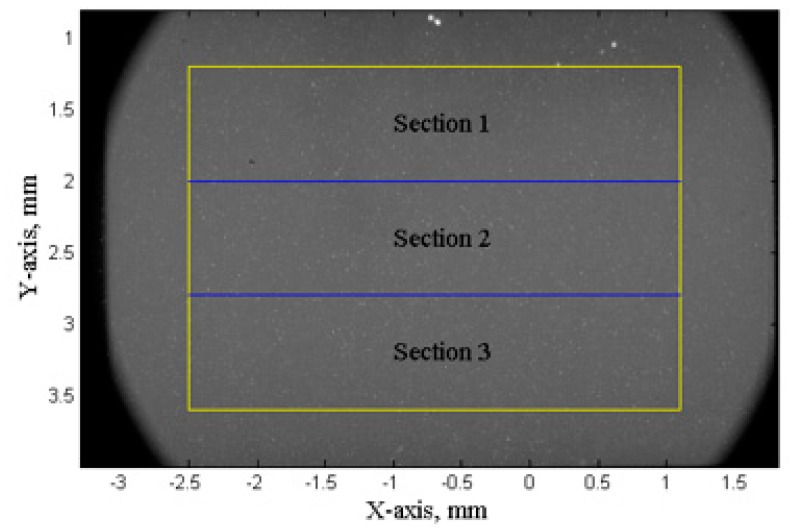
A typical X-ray image of the SCR and three sub-regions where data analysis was made.
